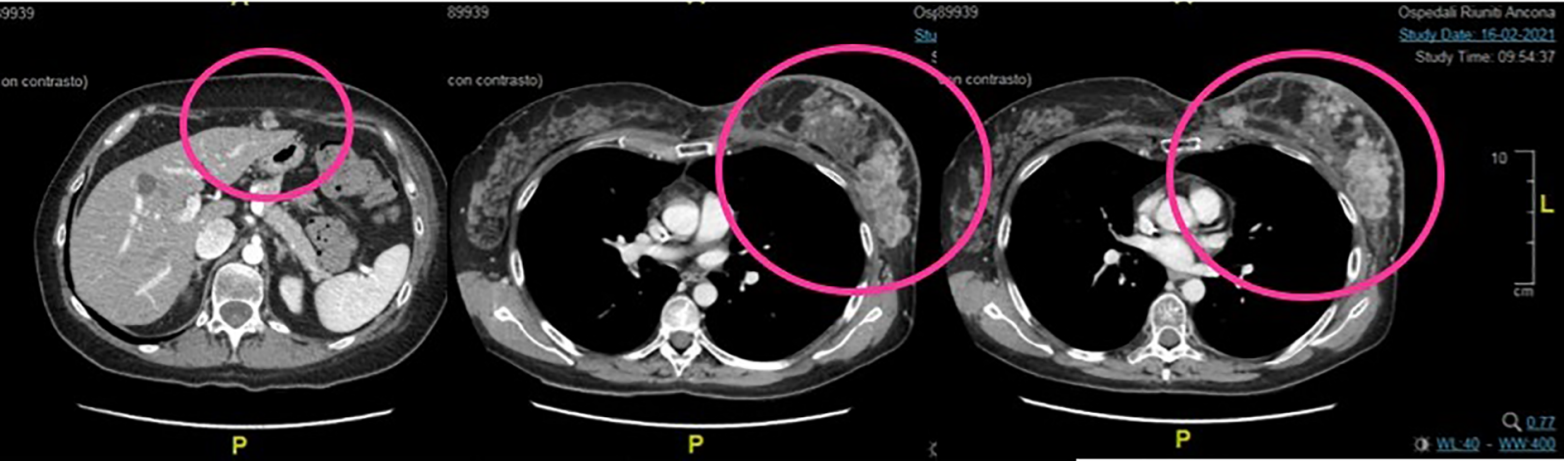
Second case. Baseline total body CT scan at the start of the treatment with TDX-d. There are evident peritoneal carcinosis, multiple subcutaneous lesions in the chest wall, and breast recurrence.
